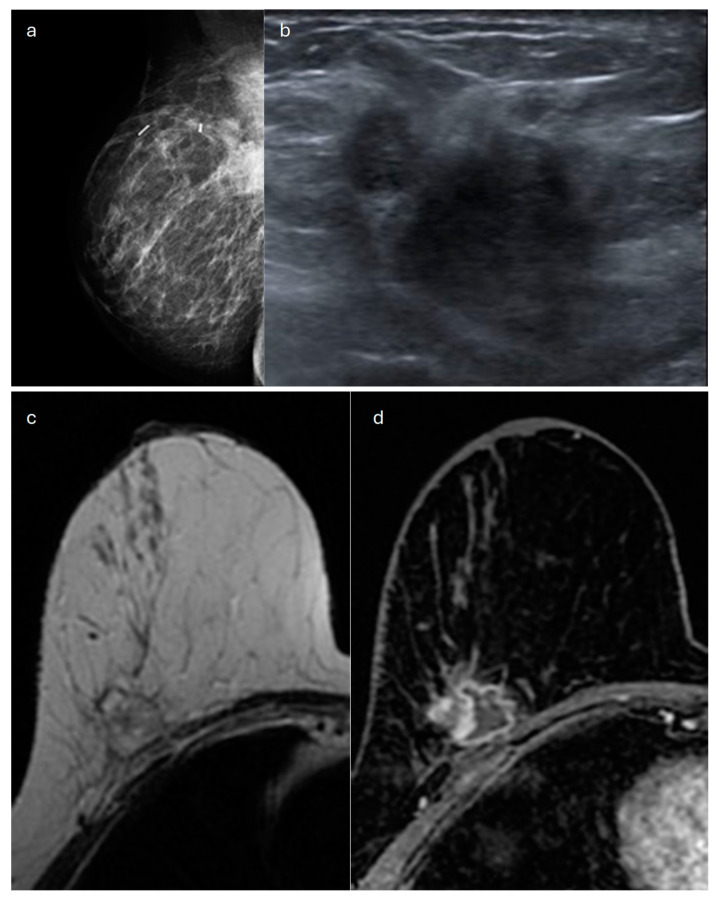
Metaplastic carcinoma in a 40-year-old patient. (a) Medio-lateral oblique mammogram of the right breast shows a high-density mass, only partially included, with indistinct margins in the upper quadrant in the posterior third. Surgical clips from a previous surgery are visible. The cranio-caudal projection is not presented because the mass was not visible on it due to its position. (b) On ultrasound, it corresponds to a hypoechoic mass with irregular margins. (c) Axial T2-weighted MRI image shows high-signal-intensity areas within the mass corresponding to necrotic components, and (d) axial 3D gradient echo T1-weighted post-contrast MRI image demonstrates a ringlike uptake.
